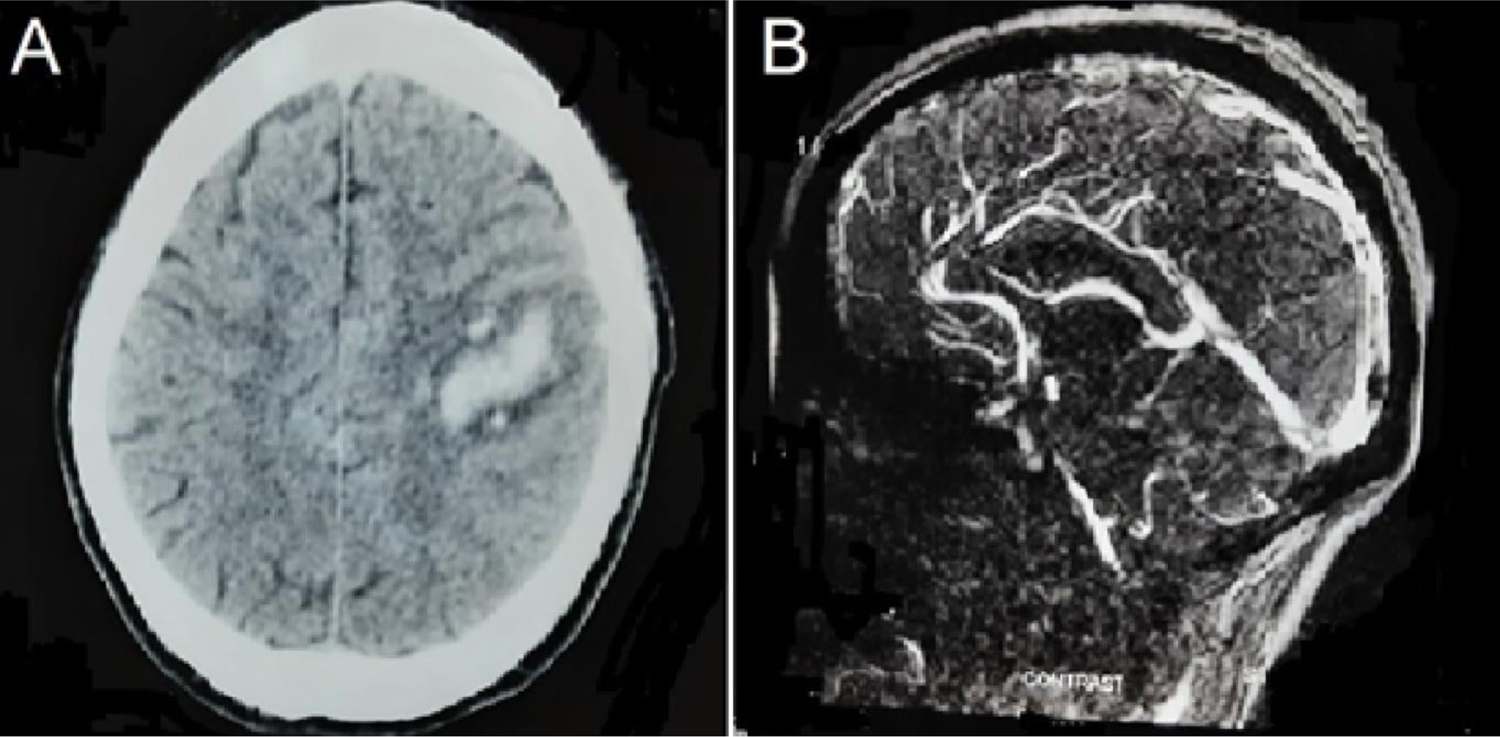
Computed tomography scan of the brain reveals an intracerebral hematoma over the left parietal region (A). Magnetic resonance venography with contrast shows a superior and inferior sagittal sinus thrombosis with multiple collaterals (B).
